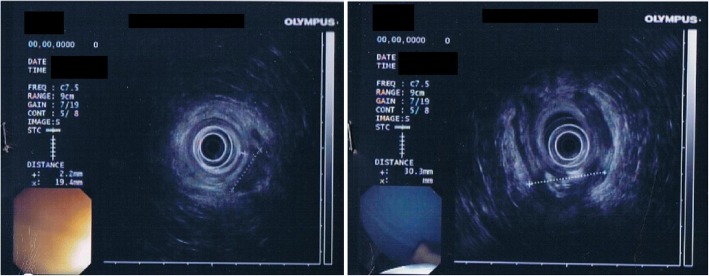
Transrectal endoscopic ultrasonography (TRUS). TRUS was performed to assess the extent of the abscess. TRUS shows normal endoscopic appearance of the rectal mucosa. Internal anal sphincter appears seamless continuity but with a thickness of about 2.2 mm. It was confirmed, in the right posterior-lateral area, the presence of an abscess 30 × 15 mm hypoechoic with hyperechoic images suggesting gas content
